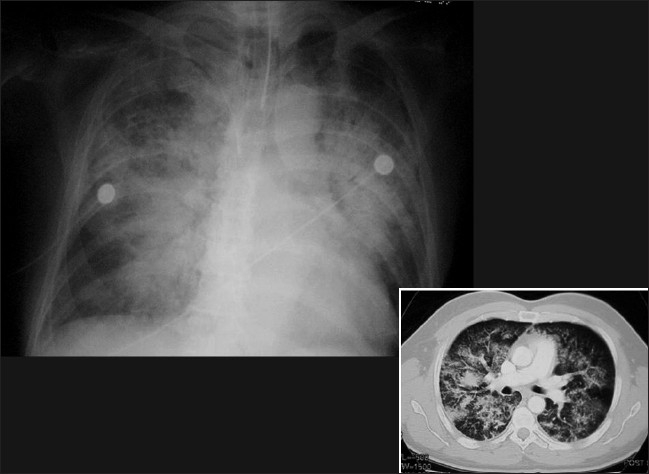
A frontal chest radiograph and axial CT show features of ‘batwing’ alveolar pulmonary edema. Chest radiographic findings include bilateral opacities that extend in a fan shape outward from the hilum in a batwing; pattern. With worsening alveolar edema, the lung opacification becomes increasingly homogenous
